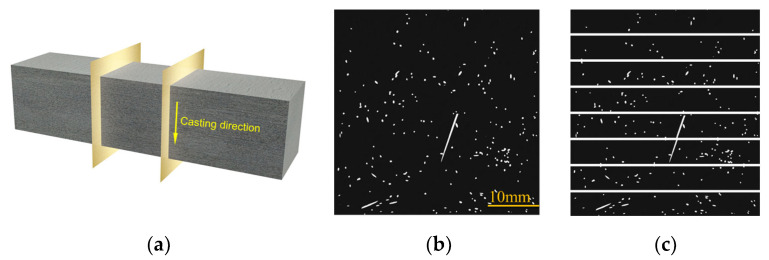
Quantitative evaluation of fiber dispersion: (a) extraction of cross-section; (b) binarized CT image; (c) segmented image for statistical analysis.
